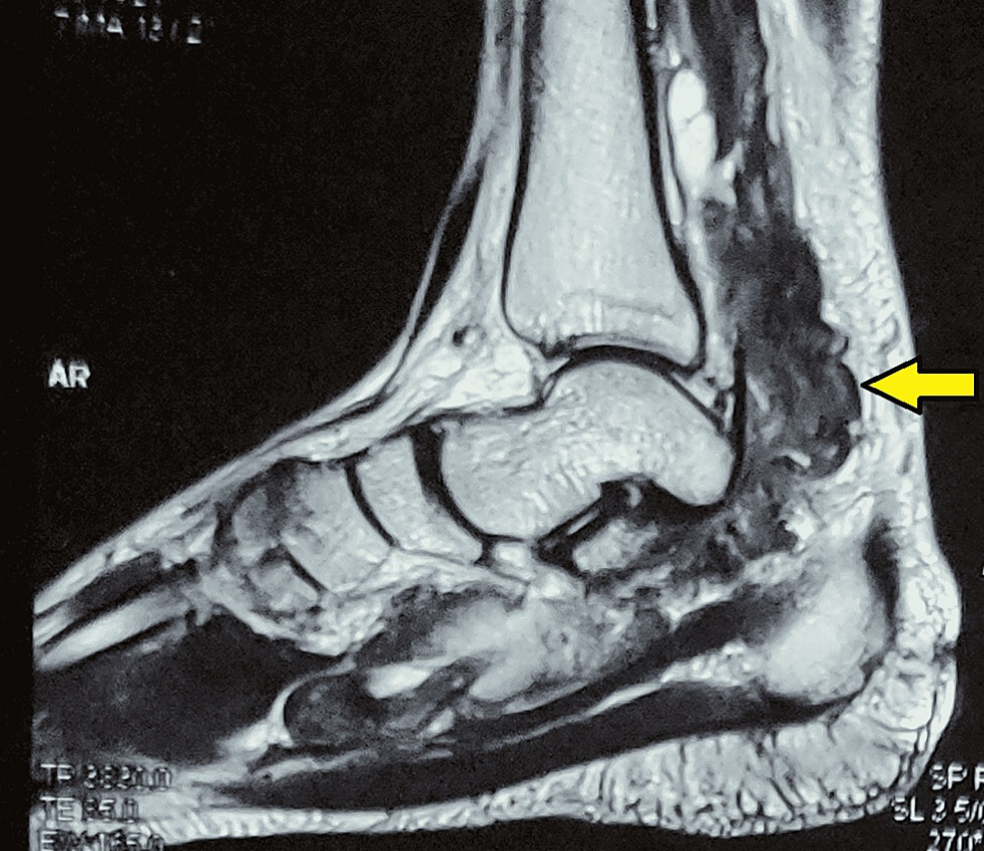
MRI sagittal section of ankle and foot Tumour extending up to midfoot
